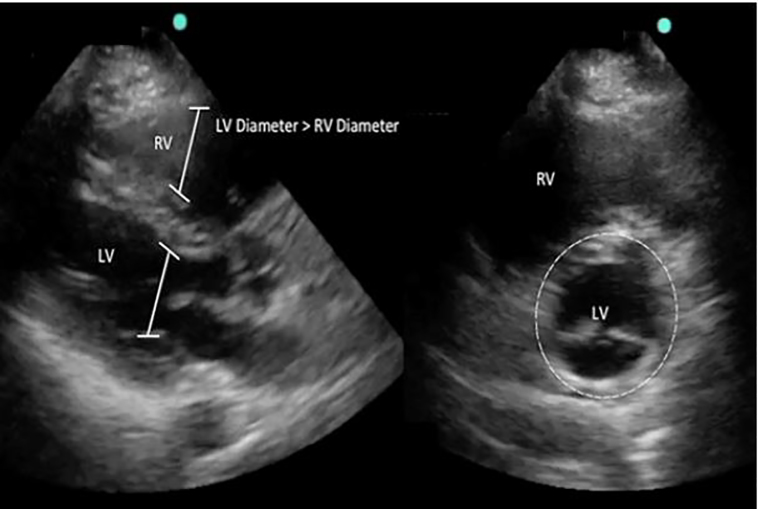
Point-of-care cardiac ultrasound post-thrombolysis. Parasternal long axis view (left) and parasternal short axis view (right) demonstrating resolution of right heart strain. *LV*, left ventricle; *RV*, right ventricle.
